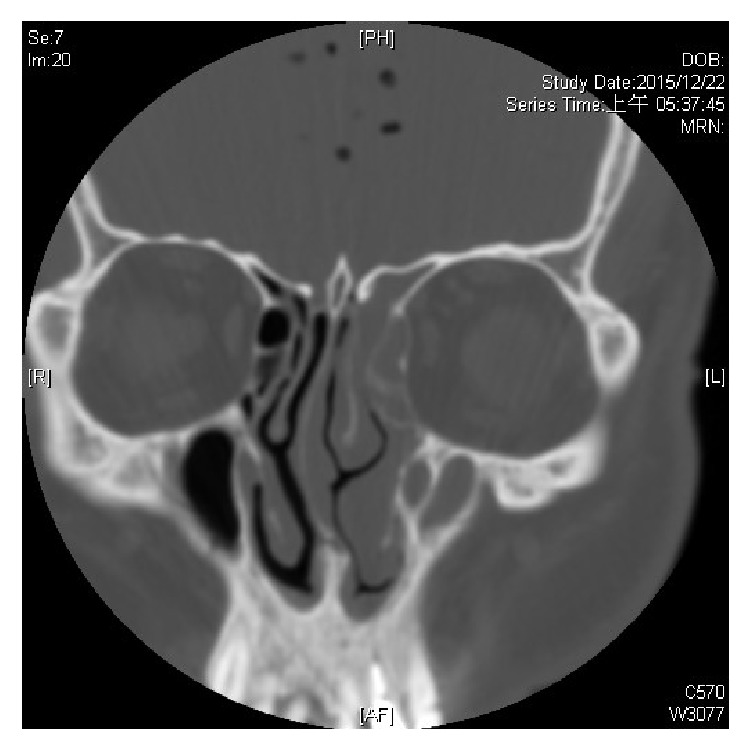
Coronal view of the CT scan reveals opacification of left ethmoid and maxillary sinus, indicating left chronic sinusitis. Note the air bubble in the left olfactory fossa, which gives us a hint of the possible site of breach in the lateral lamella of cribriform plate on patient's left side.
